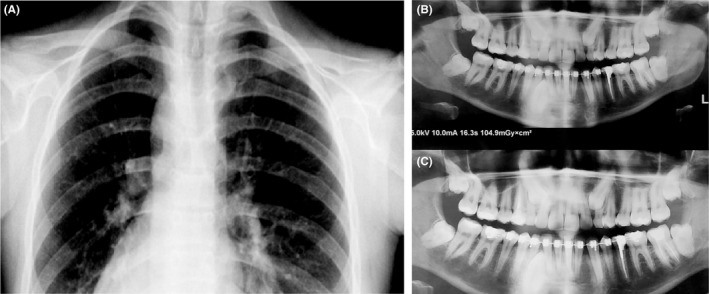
The radiograph images of bone clavicles and teeth in the member II:1. Hypoplastic form and incomplete growth of clavicle bones (A). Supernumerary teeth and the late eruption of permanent teeth (B, C). The patient was under orthodontic therapy at the time
